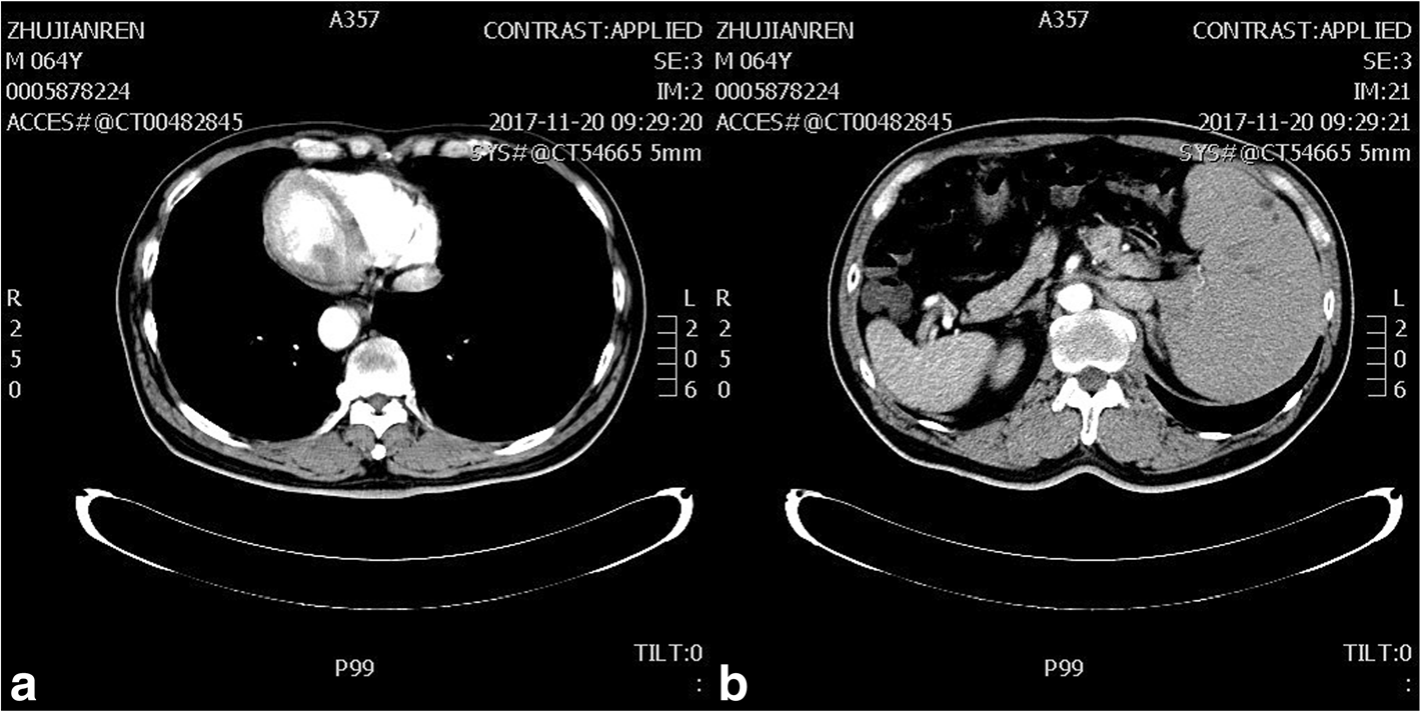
The preoperative computed tomography scan showed a complete transposition of the thoracic and abdominal viscera. a Mirror-image dextrocardia. b The liver was located on the left side of the abdomen
